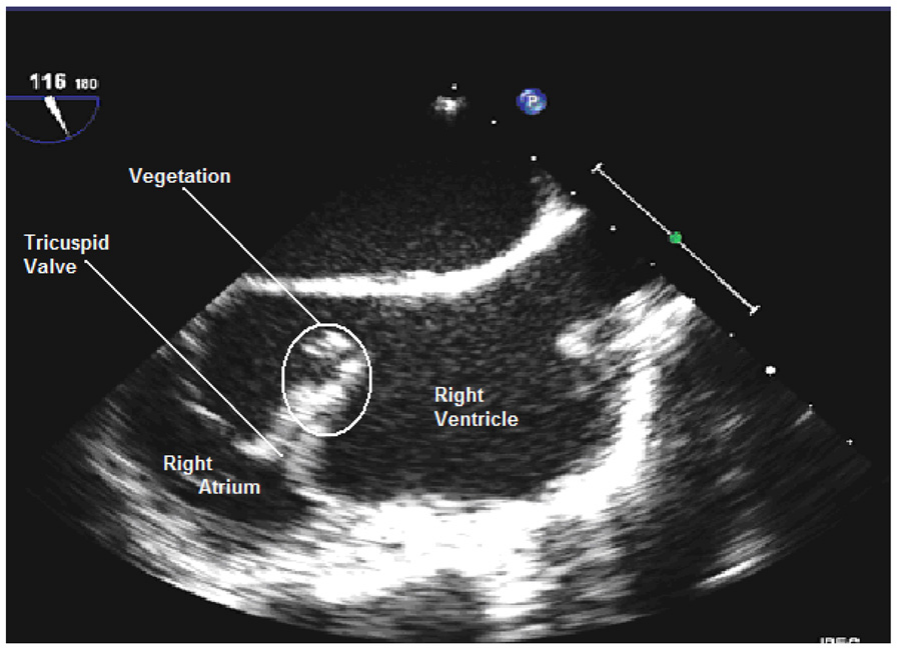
Transesophageal echocardiogram image of tricuspid vegetation. After initial imaging of the vegetation by a transthoracic echocardiogram, a transesophageal echocardiogram was performed to rule out an associated root abscess and in anticipation of a preoperative work-up.
